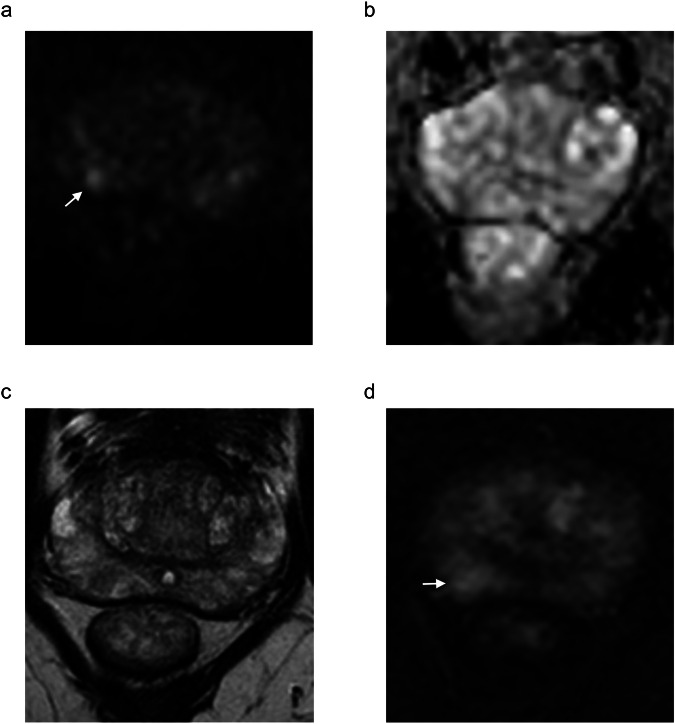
A 60-year-old man showing a PSA level of 4.45 ng/mL. PI-RADS 3 upgraded to 4 category was attributed to a lesion in the right mid-gland posterior-lateral peripheral zone showing marked hyperintensity on the high b-value image (arrow in a), mild hypointensity on the ADC map (b), mild hypointensity on T2-weighted imaging (c) and focal early contrast enhancement (arrow in d). Target biopsy cores included high-grade prostatic intraepithelial neoplasia, atypical small acinar proliferation, and chronic inflammation. Biopsy strategy 7 would have avoided biopsy as the lesion showed an ADC value of 1.11 × 10^−^^3^ mm^2^/s, corresponding to a high diffusion level (H-DL)
